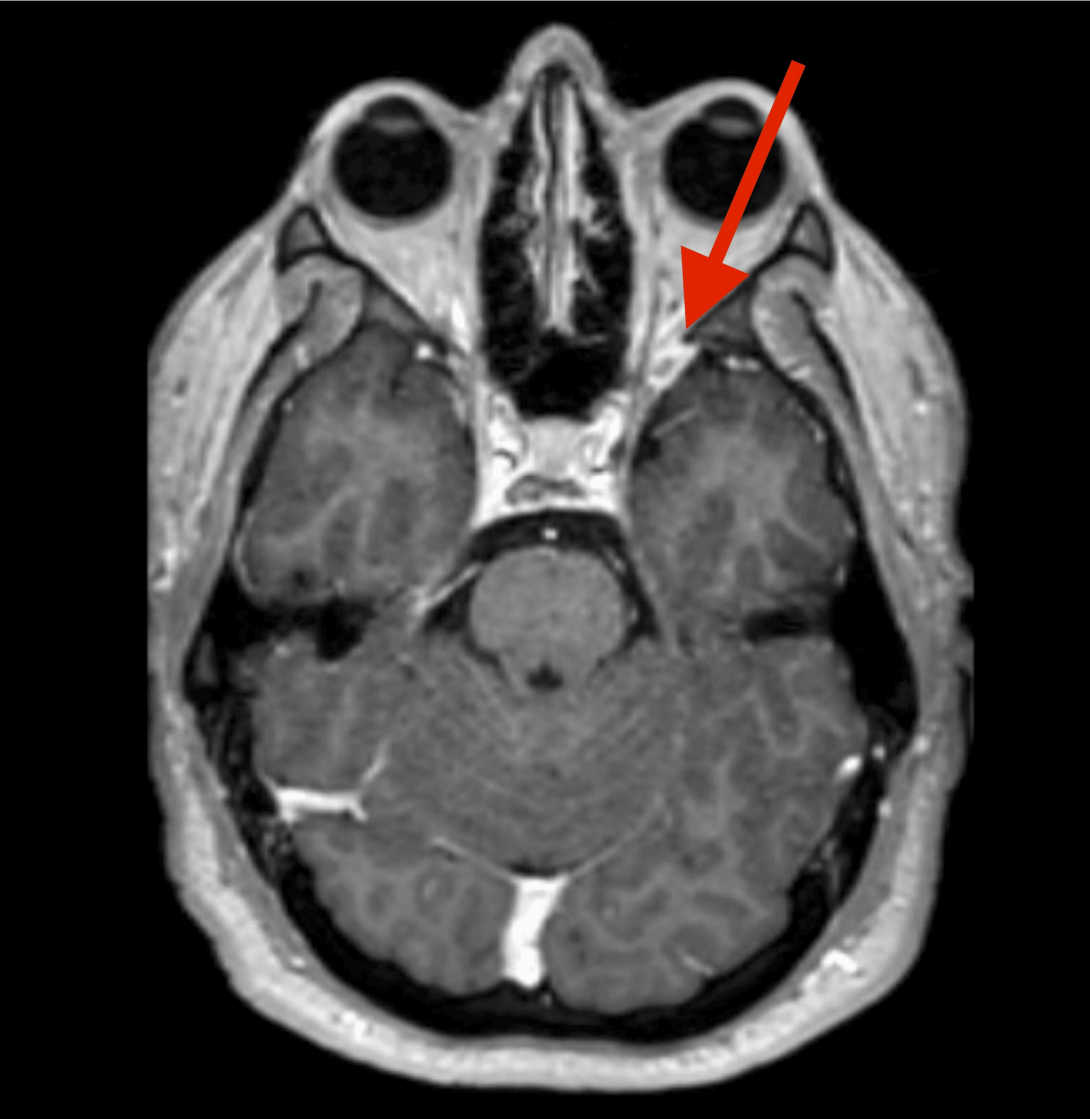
Axial T1-weighted post-contrast MRI demonstrating a homogeneously enhancing dural-based lesion along the left cavernous sinus with mild sphenoid-wing extension, initially interpreted as a sphenoid-wing meningioma.
